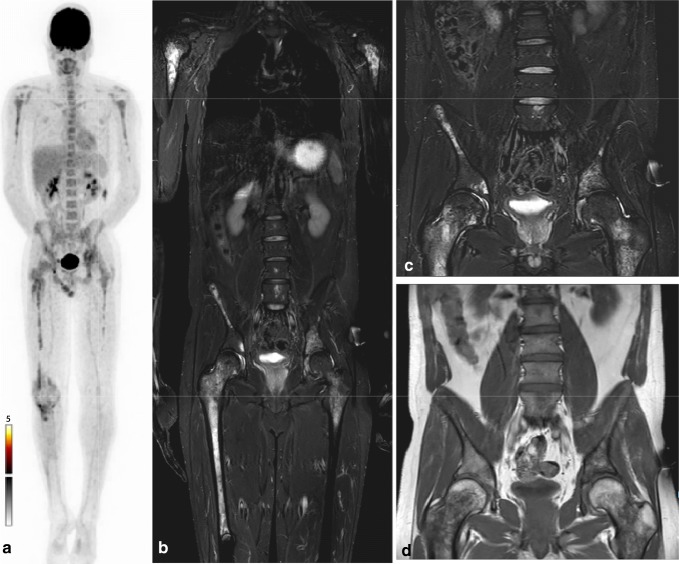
False-negative lesions on ^18^F-FDG PET-CT with widespread hematopoietic bone marrow activity. A 23-year-old man diagnosed with localized Ewing sarcoma of the right proximal tibia. Images obtained 6 months after initial treatment (6 × VIDE, surgery, 8 × VAI), at this time undergoing second-line chemotherapy because of recent distant metastasis. a^18^F-FDG PET-CT with symmetrical ^18^F-FDG uptake in the axial skeleton and proximal extremities. This was classified benign (negative) owing to anemia or recent chemotherapy. b T1-weighted short tau inversion recovery (STIR) MRI images with multifocal metastatic lesions throughout the whole axial skeleton. c STIR images with several skeletal metastases in the left and right ilium and fifth lumbar vertebral body. d T1-weighted turbo spin echo (TSE) images with several skeletal metastases in the left and right ilium and fifth lumbar vertebral body
